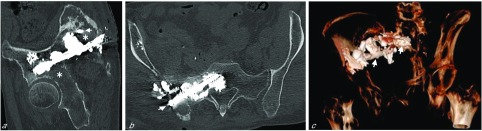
(a) Oblique sagittal reformatted view of post-procedure CT (bone window, slice thickness 1 mm) of the pelvic bones after inserting screws and first step of cementoplasty. The cross section of the proximal part of the sacral screw is visible (small arrow). After this procedure, two large osteolytic areas compromising the whole stability of the osteosynthesis remained, one in the right acetabulum and the other in the right posterior iliac wing with no cement continuity between the two screws at that step (asterisk). (b) Oblique coronal reformatted view of post-procedure CT (bone window, slice thickness 1 mm) of the pelvic bones after inserting screws and first step of cementoplasty shows the sacral screw (small arrow) anchored into the bone with cementoplasty and a slight extravasation of bone cement in soft-tissue. (c) 3D oblique anterior reformatted view of post-procedure CT shows the whole osteosynthesis with the two screws (small arrows) surrounded by cement.
